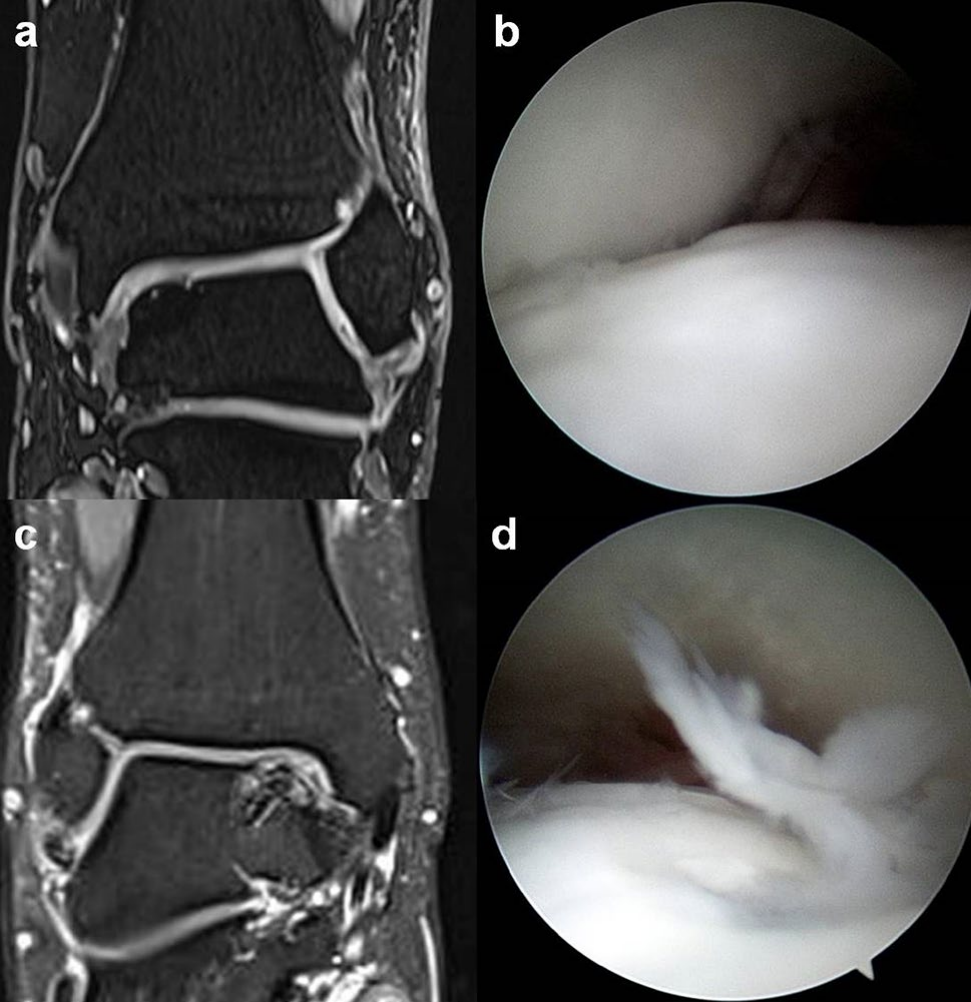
(a) The postoperative MRI of an 18-year-old female patient with bone fragment union shows normal congruity of the fixed osteochondral fragment without any cysts underneath the fragment. (b) The second-look arthroscopic image shows smooth, pearly, hyaline-like cartilage of the osteochondral fragment, which is well integrated with surrounding cartilage. (c) The postoperative MRI of a 21-year-old male patient with bone fragment nonunion shows incongruity of the fragment with irregular cartilage and cyst underneath the fragment. (d) The second-look arthroscopic image shows severe fibrillation and flap tearing of the cartilage.
